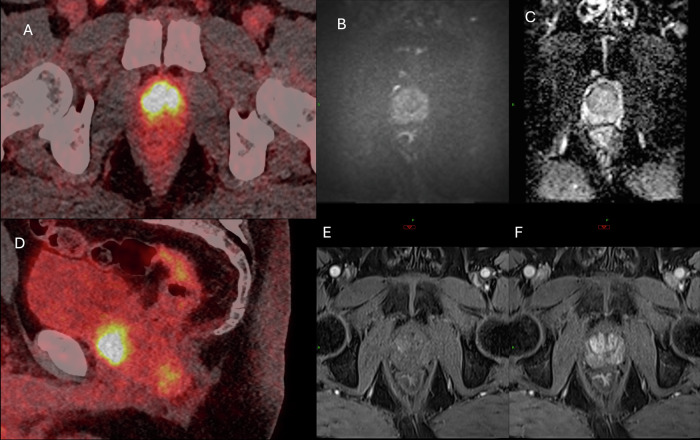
MRI-negative and PSMA-positive prostate malignancy. 56-year-old patient with PSA 11.1 ng/mL, PSA density 0.38, prostate 28 mL. MRI report of PI-RADS 2. Biopsy showed Gleason 3 + 4 prostate malignancy. PSMA PET/CT demonstrated a 3.6 cm intensely PSMA-avid area in the apex of the prostate and in the transitional zone, SUVmax 13.9. Intensely PSMA-avid prostate malignancy centred in the transitional zone. A, D (Fused transverse and sagittal) PSMA PET/CT. Negative multiparametric prostate MRI, homogeneous gadolinium enhancement pattern (E, F), axial diffusion-weighted imaging b1400 (B) and axial apparent diffusion coefficient map (C) showed no MRI-visible lesion corresponding to the PSMA-avid lesion
